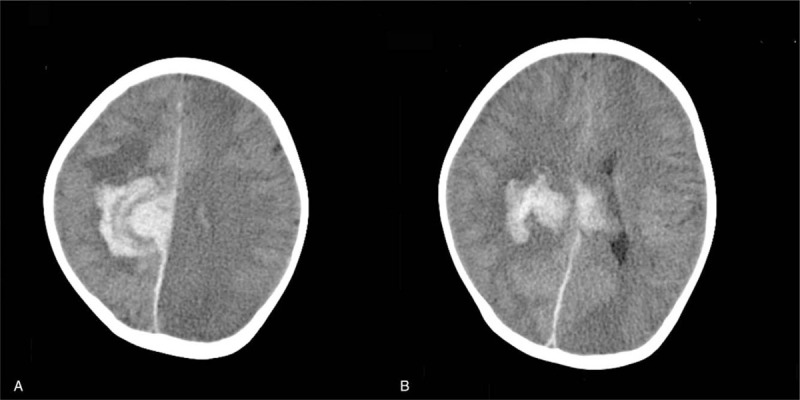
CT on the 7th postoperative day. Note: There are massive high-thickness tracker in the anterior parietal lobe-ventricular body on the right side, and low-density edema gumshoe on the surrounding brain parenchyma. The space-occupying effect is obvious. The right lateral ventricle and the midline structure are compressed and shifted to the left. Both sides High-density shadows were seen in the later horn of the lateral ventricle. The left forward lobe and parietal occipital part show flaky low-density reduction areas.
